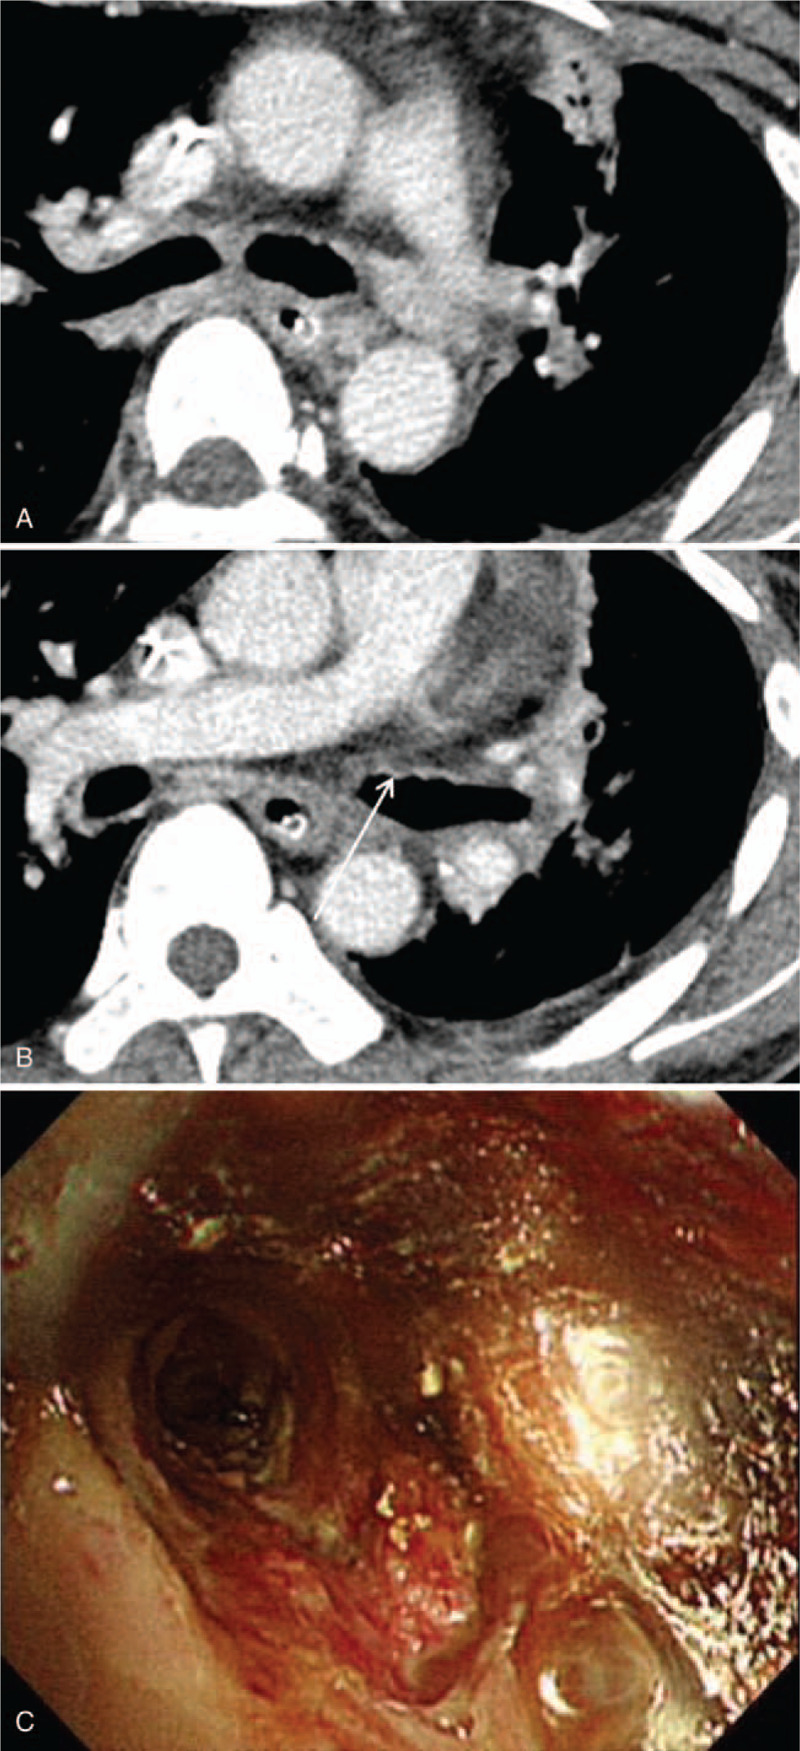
Chest CT findings of airway injury 1 month post acid ingestion. A, B. Irregular surface with wall thickening of the left main bronchus is shown on chest CT C. Multiple white patches and erosions are shown on the left main bronchus.
